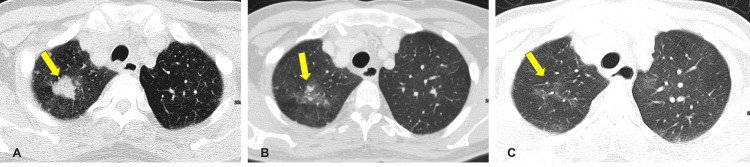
(A) Computed tomography from August 2020 (approximately eight months after discounting sunitinib): the reappearance of bilateral pulmonary nodules, largest at the right upper lobe measuring 3 cm. (B) Computed tomography repeated three months later showed a significant regression of the right upper lobe metastatic lesion. (C) Most recent computed tomography revealing complete resolution of the metastatic pulmonary nodules.
